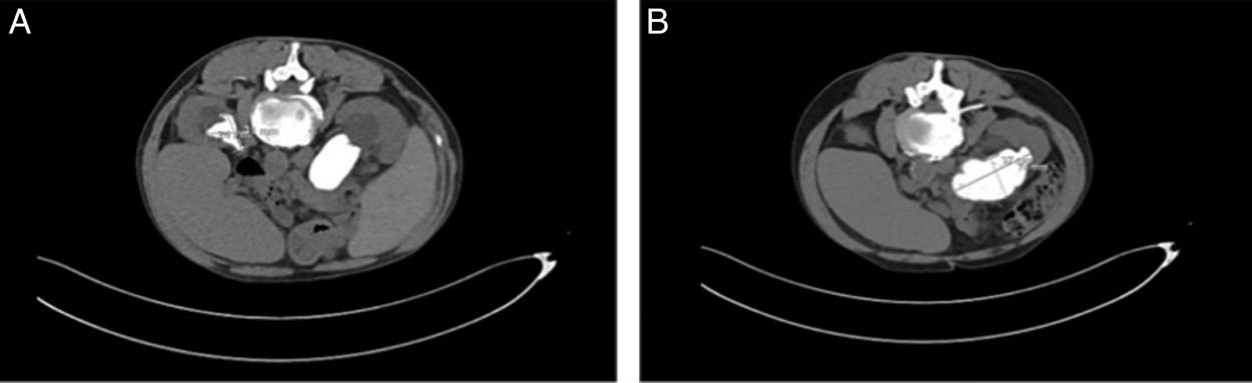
Axial view computed tomography scan taken upon initial presentation.
